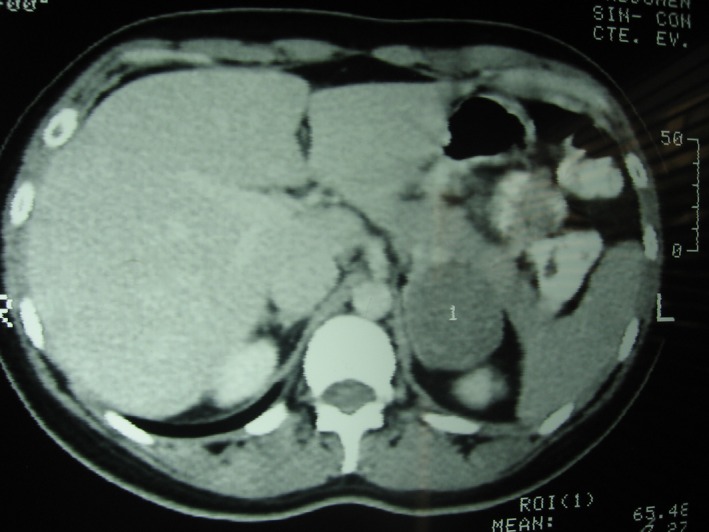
CT scan showed a slightly enhanced tumor in the left adrenal gland.
